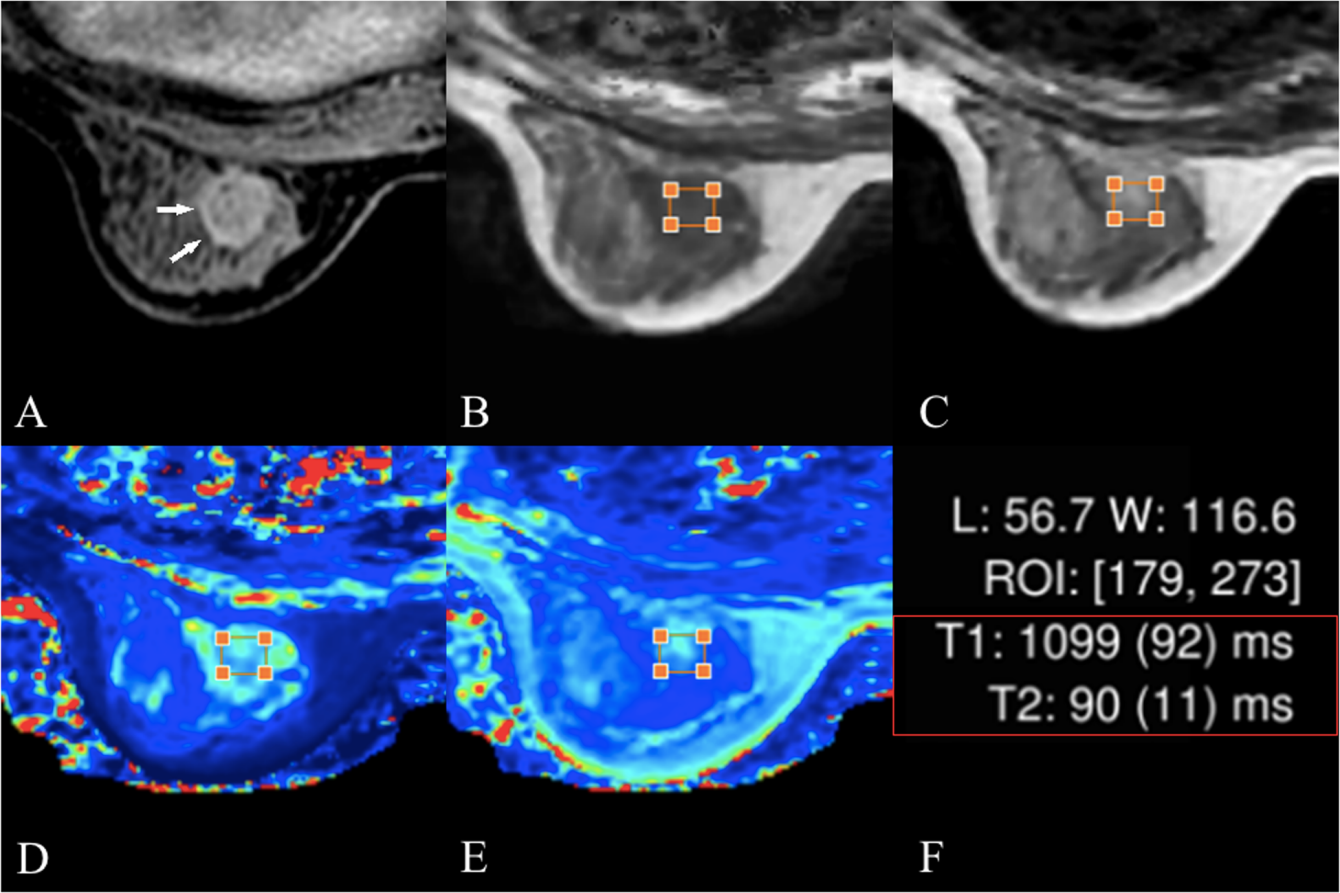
Female, 50 years old, fibroadenomas. a the lesion is shown using white arrows in the perfusion-enhanced image; b and c, T1-, T2-weighted images obtained from SyMR respectively I; d and e T1 and T2 maps, respectively. The T1 and T2 values are shown in f
